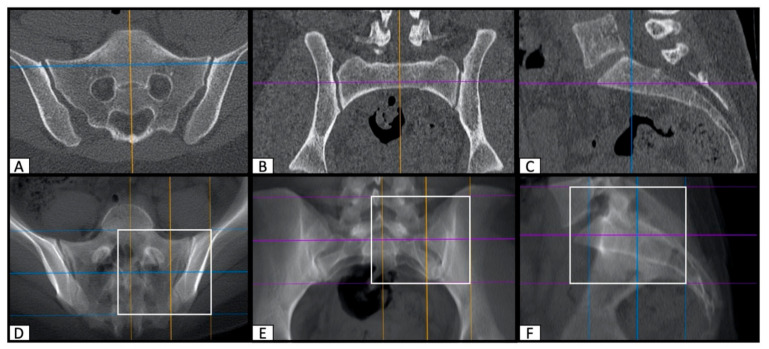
Symmetric orientation of the CT scans using the multi-planar reconstruction function of Osirix MD in axial and coronal orientations (A,B) to create an exact lateral view (C). Marking the region of interest (white box) and fusion of this area by means of the thick slab function (D–F) of OsiriX MD to create a two-dimensional exact lateral image of the sacrum (F).
